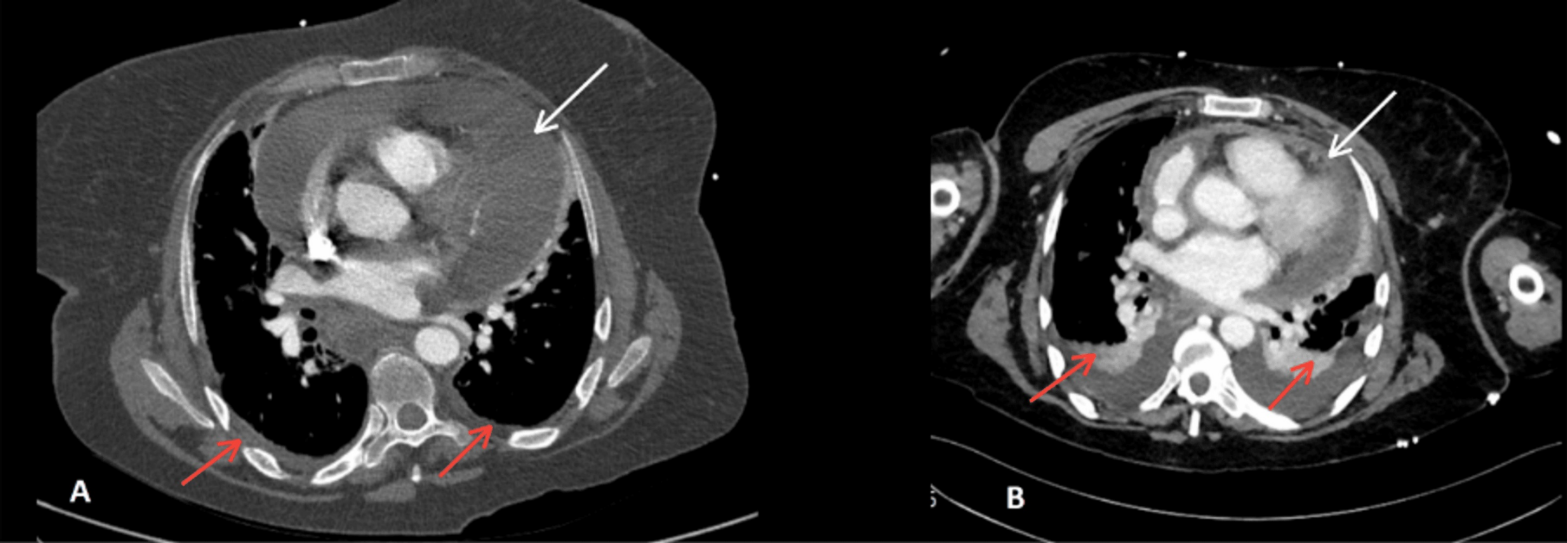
Chest CT angiograms - vascular windows (A) CT aortogram on Day 1 showing a large pericardial effusion (white arrow) and minimal bilateral pleural effusions (red arrows). (B) CT pulmonary angiogram on Day 2 showing interval reduction of the pericardial effusion (white arrow) with mild pleural effusions (red arrows).
